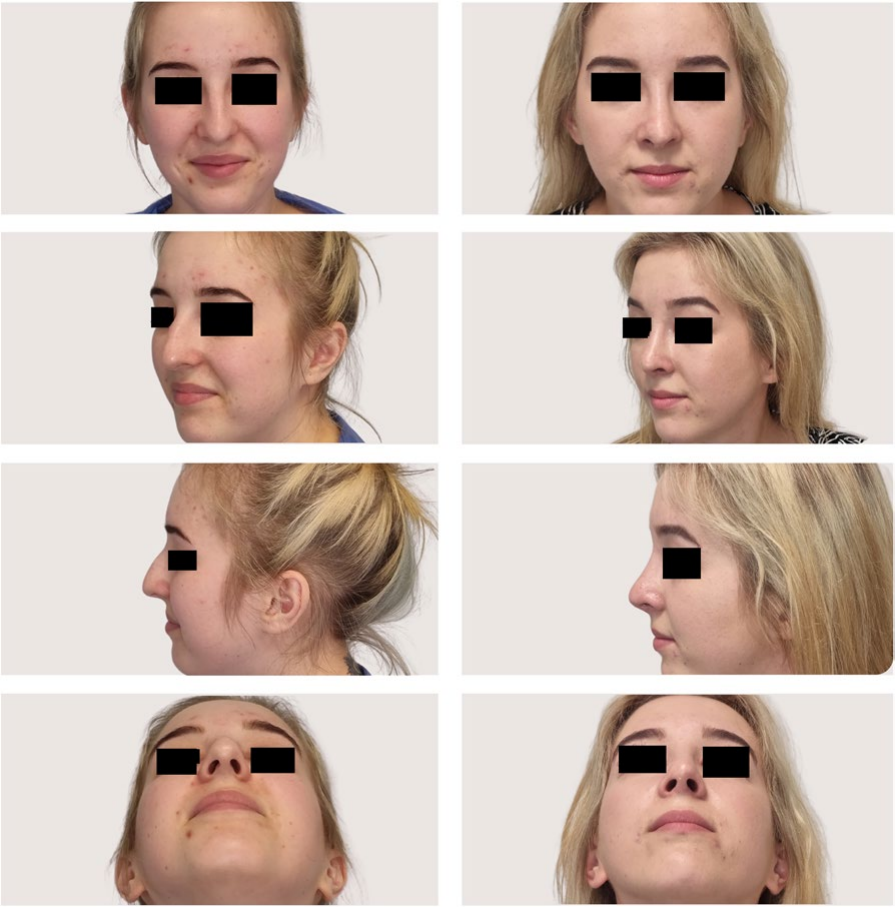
CT-assisted precision rhinoplasty in patient C desiring classical subdued aesthetic outcome
